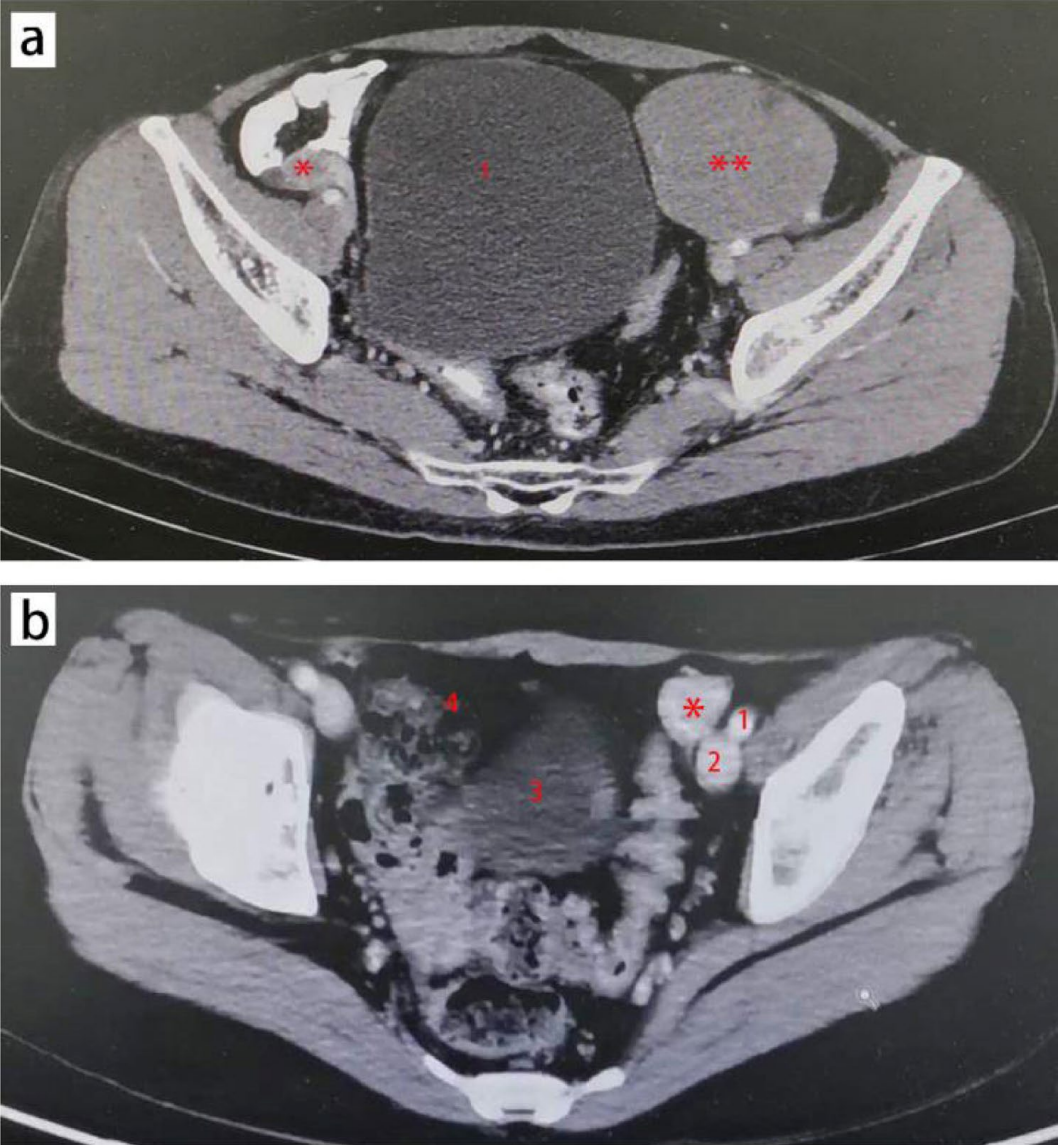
Preoperative CT imaging of the pelvis of case 1 (a) and case 2 (b). (a) left side mass (showed with ): 7.7 × 6.4 cm, located near the anterior abdominal wall and bladder (showed with 1); right side mass (showed with *): 2.2 × 2.0 cm, a residual testicular structure, located in the right inguinal canal. (b) left side mass (showed with *): 3.4 × 2.0 cm, located near external iliac artery (showed with 1) and external iliac vein (showed with 2); 3: Bladder; 4: Fibrous scar tissue caused by last surgery.
